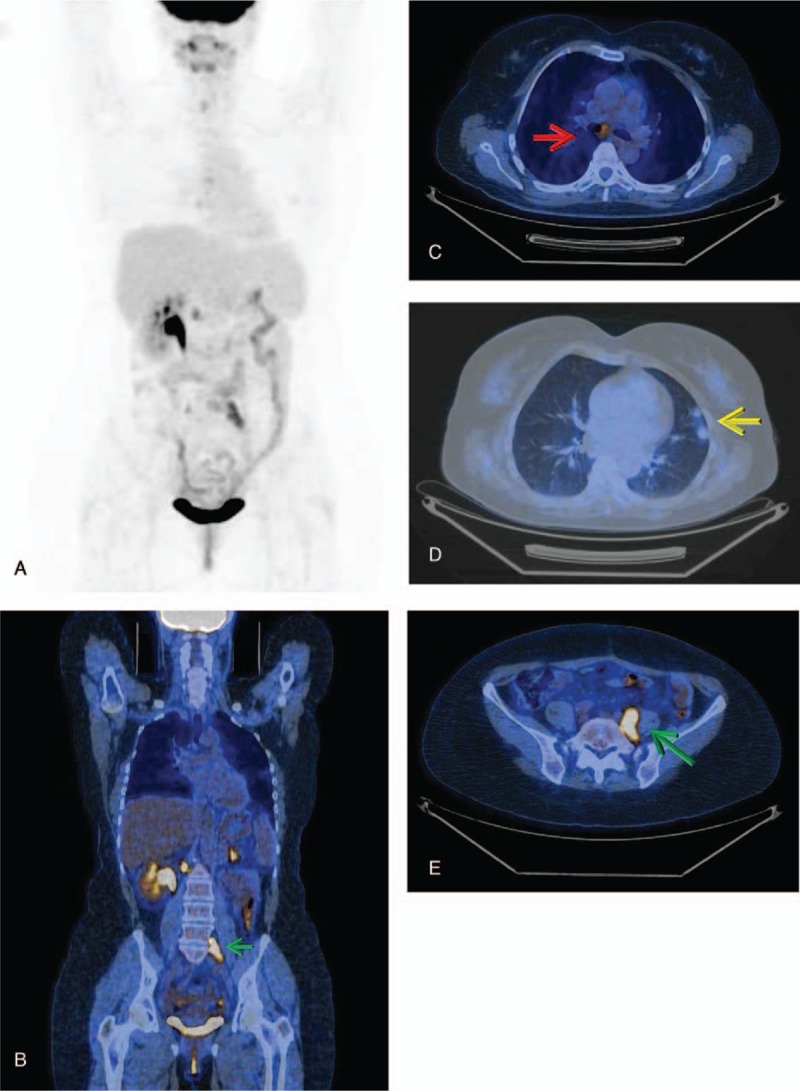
18F-FDG PET/CT (A) MIP, (B) coronal, and (C-D-E) axial fusion images detected disease progression with an increased uptake of radioactive tracer in several lymphnodes in subcarinal (red arrow) and para-aortic sites (green arrows). Two lung lesions (yellow arrow) showed an increased uptake of 18F-FDG. Left kidney is not visible because of previous left nephrectomy.
